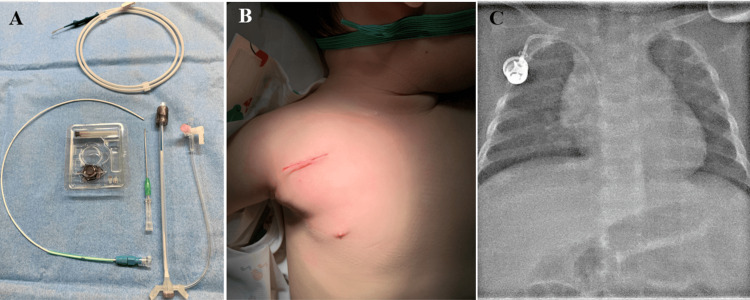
Procedural details. (A) Photograph of the CELSITE small port, 5 Fr Anthron PU catheter, peel-away sheath, 18 G needle, and 0.035” wire. (B) Chest images after the central venous port implantation in a two-year-old patient. (C) Chest radiography following the procedure.
